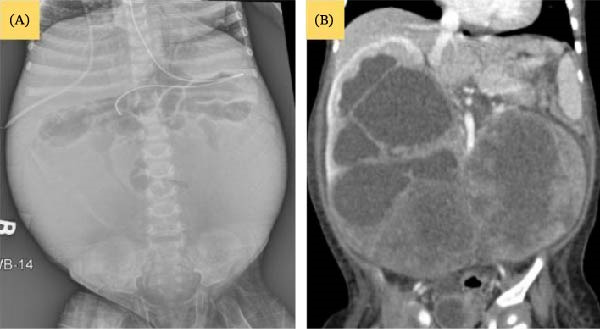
(A) Frontal abdomen radiograph shows central and superior displacement of the aerated bowel loops. Large soft tissue density is noted in the right and left abdomen, which is related to the enlarged horseshoe kidney. (B) An enhanced CT scan of the abdomen in coronal view shows bilateral renal enlargement with cross‐fusion of the lower renal poles, representing horseshoe kidney. Bilateral lower renal poles demonstrate large heterogeneous masses. Right hydronephrosis is also appreciated (Case 2).
